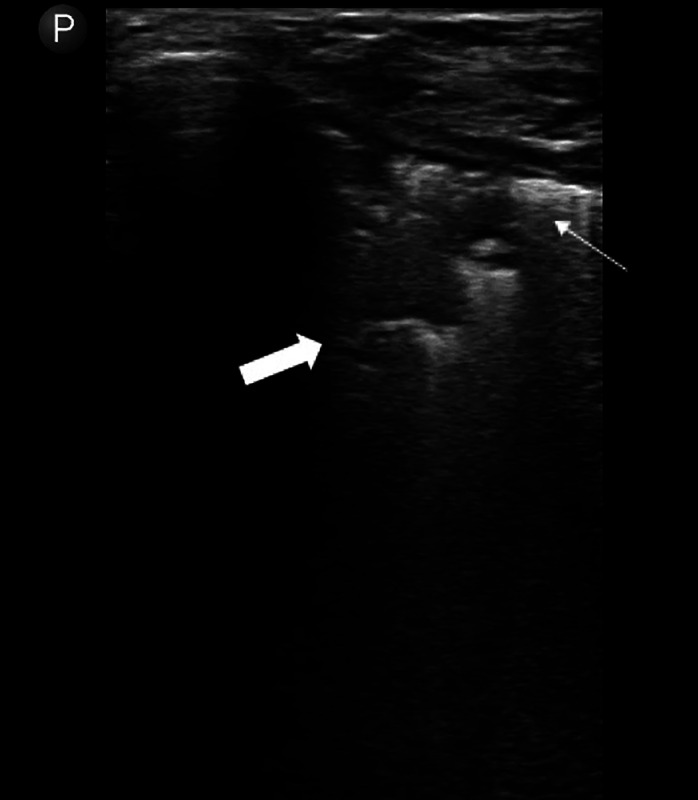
Apical consolidation (thick arrow) adjacent to pleural line (thin arrow) in 30-year-old patient with 3 months productive cough, fever, and weakness. Sputum smear was positive for acid-fast bacillus. Lung ultrasound demonstrated bilateral upper lobe consolidations and absent lung sliding in bilateral anterior fields. The patient was treated for pulmonary tuberculosis.
